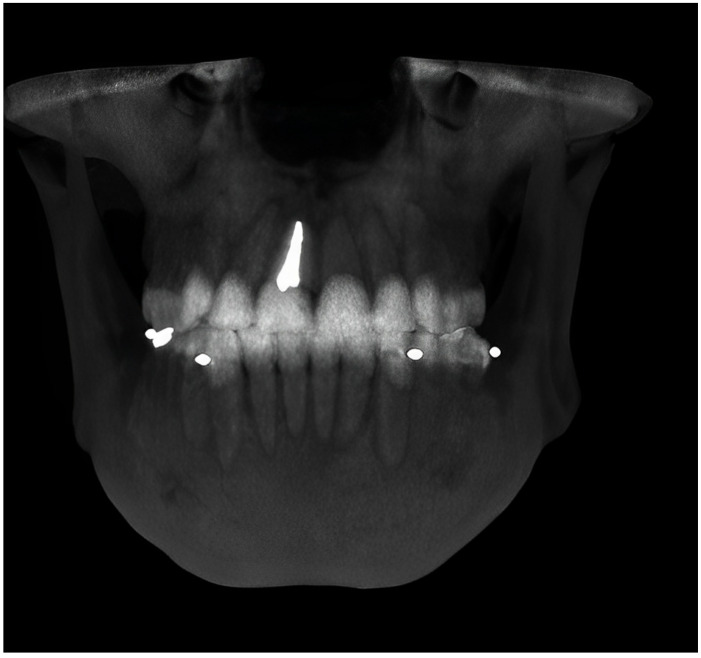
The post-CBCT was executed in maximum intercuspation (MI).
